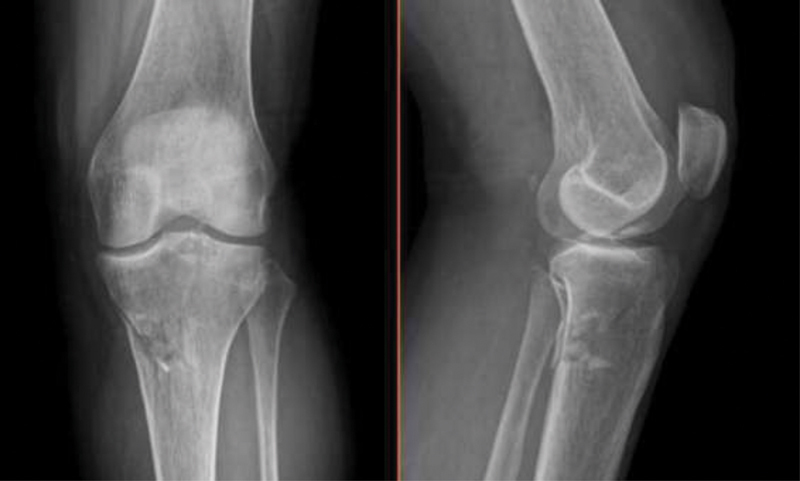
Anteroposterior and lateral radiograph in the emergency room.
